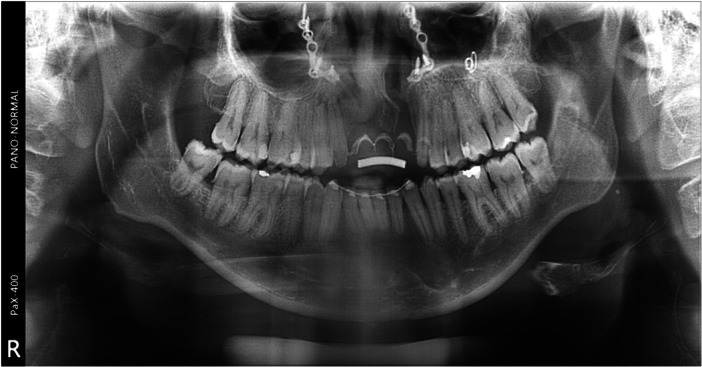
A panoramic radiograph after 36 months of follow‐up, showcasing the previous surgical repair of bilateral cleft lip and palate with oronasal fistula.
